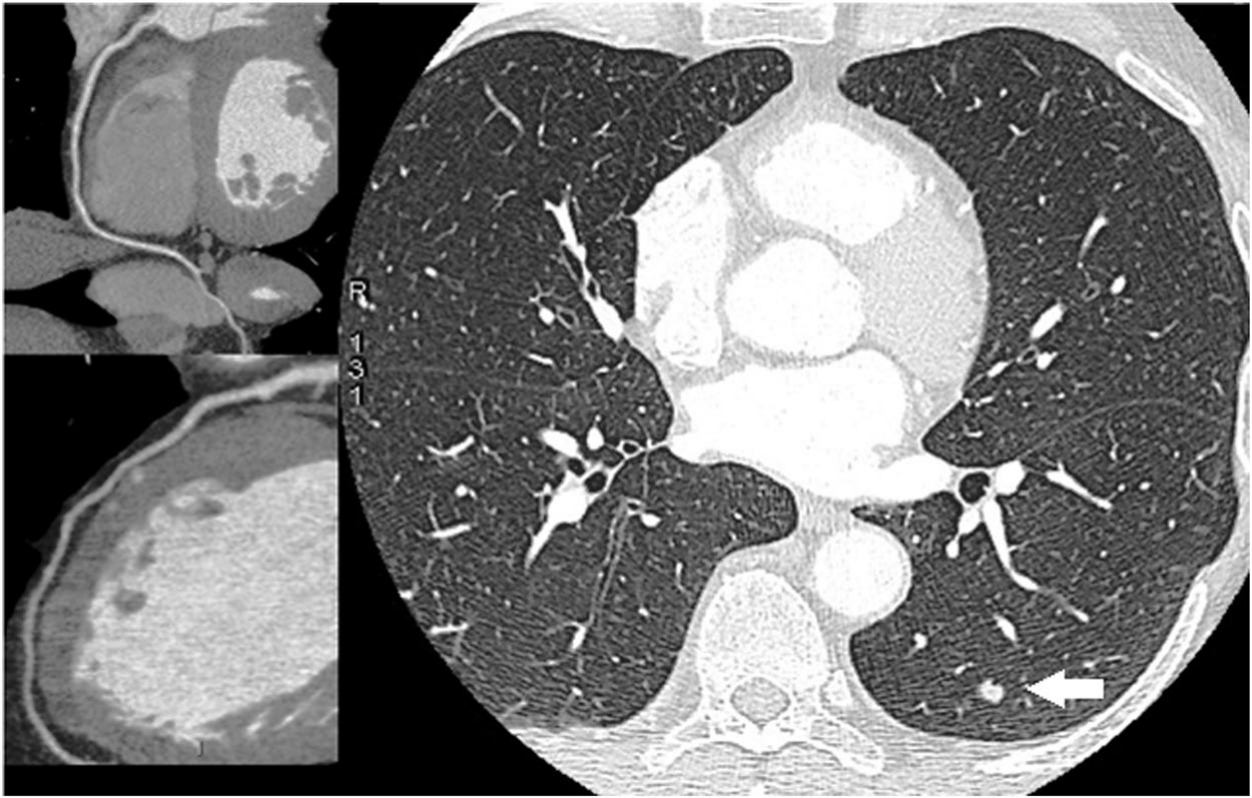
A case of coronary artery disease and lung cancer screening. Ultra-low-dose CT images of the lungs showed a 2 mm pulmonary nodule (*white arrow*) in the left upper lobe (right panel). The cardiac CT revealed normal right coronary artery (left upper panel) and left coronary artery (left lower panel).
